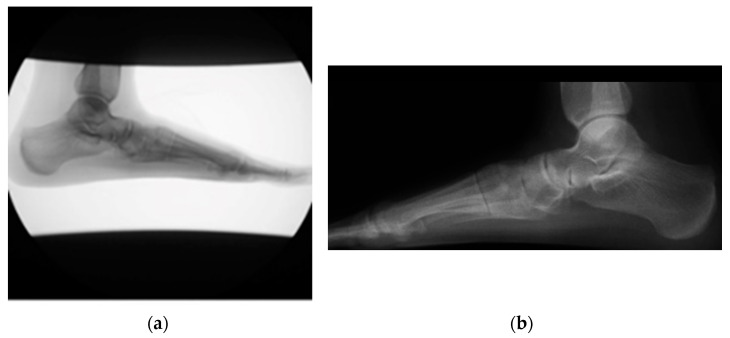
(a) An input X-ray image example of the right foot. The radiograph shows a white background, and the foot is not in the center of the image. (b) The same input image example from (a) after implementing image preprocessing (black background, cropping, re-centering, horizontally flipping, brightness, and contrast equalization).
